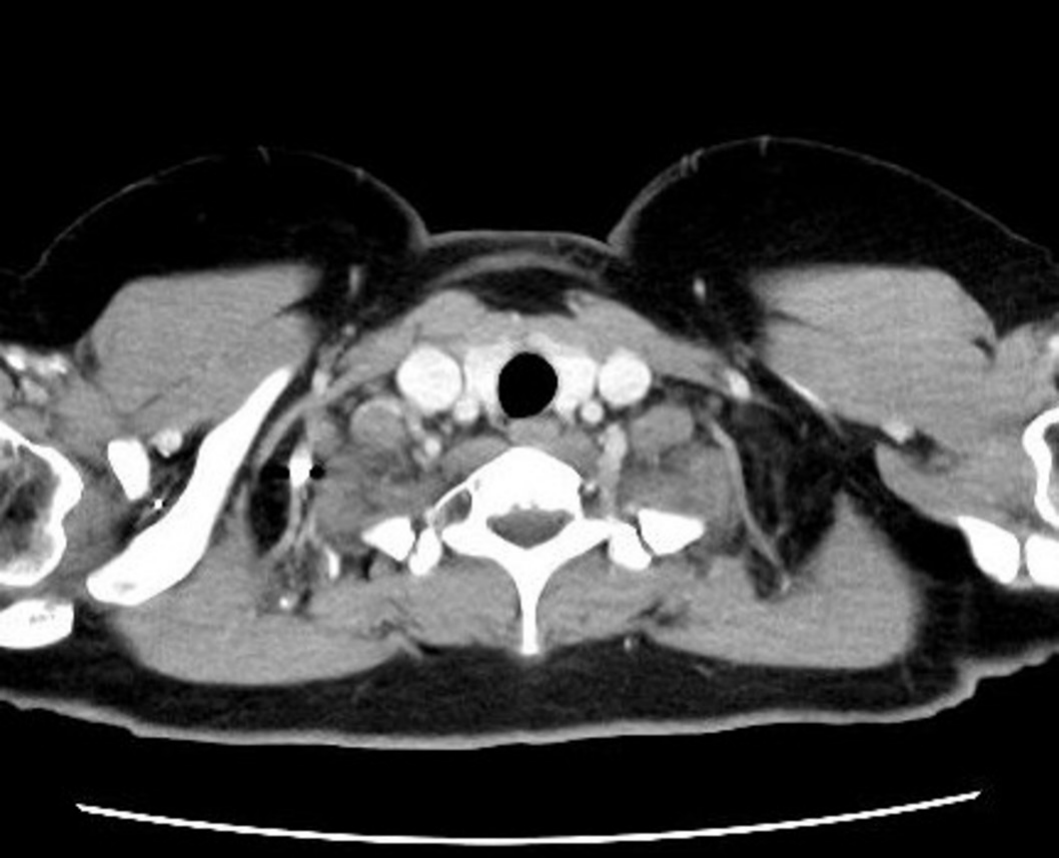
Contrast‐enhanced CT showed resolution of the right cervical lymph node.
